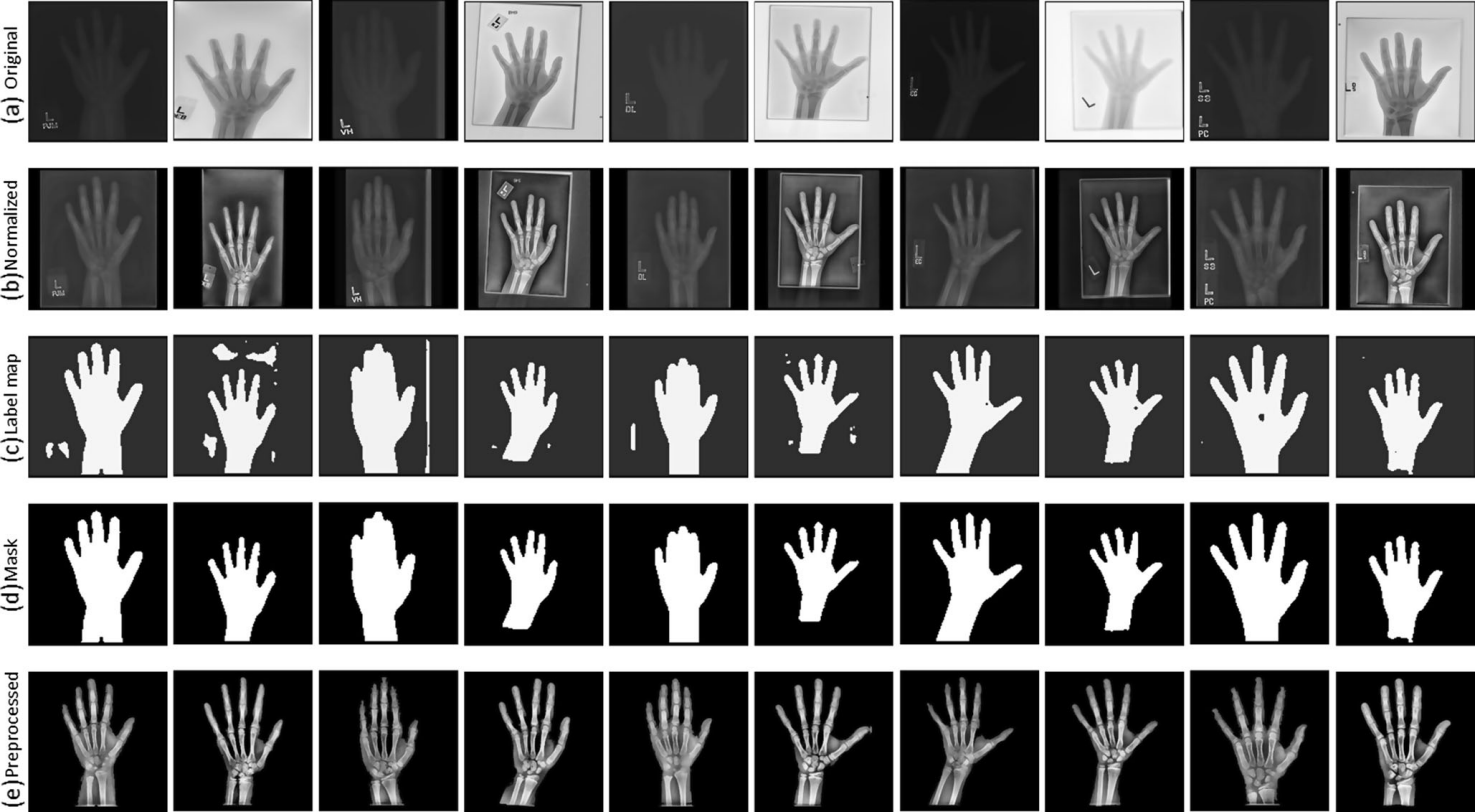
Ten examples at each stage of preprocessing as described in the “Preprocessing engine” section *a* Input radiographs. The images have been transformed to a square shape for consistent layout. *b* Normalized images with consistent grayscale base and image size. *c* Label maps of hand (*white*) and non-hand (*black*) classes. *d* Generated masks for segmentation. *e* Final preprocessed images.
